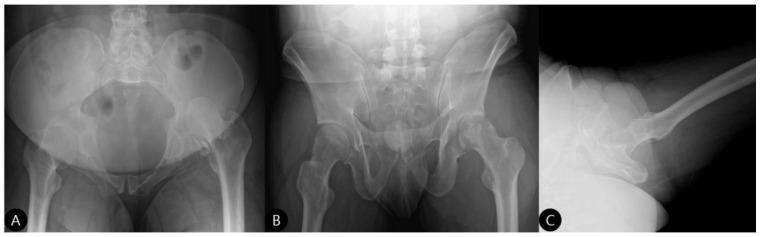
Initial radiographs upon admission (2 October 2023). (A) Pelvic outlet, (B) inlet, and (C) cross-table lateral views demonstrating a left acetabular posterior wall fracture with posterior hip dislocation.
